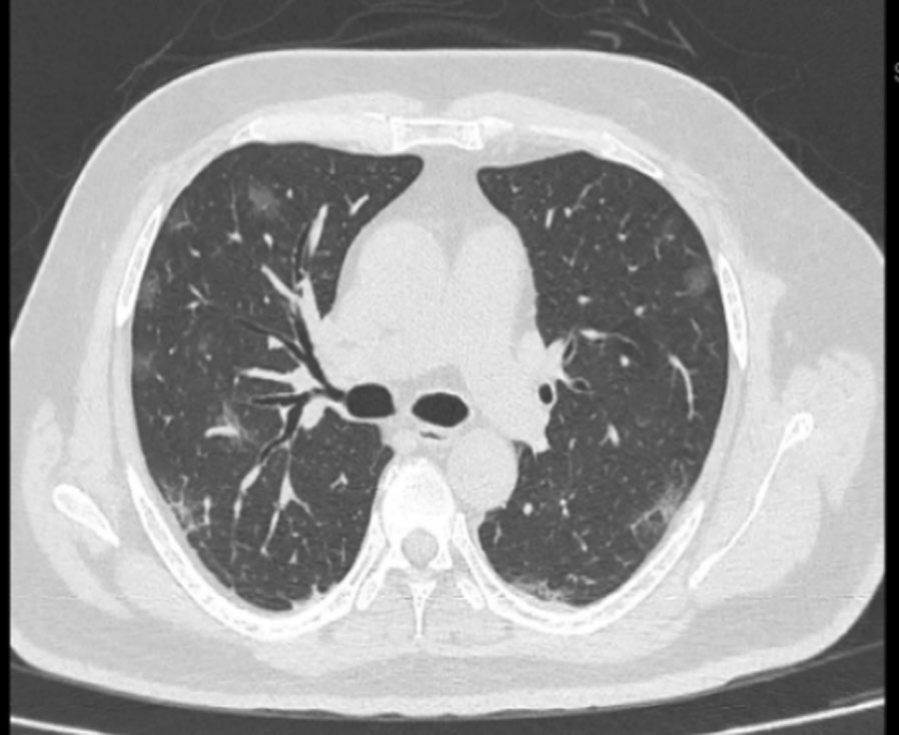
Axial CT image indicating mild disease severity (CTSS 5). Axial CT image shows GGO in bilateral upper and lower lobes mainly in subpleural and peripheral location with CTSS 5
